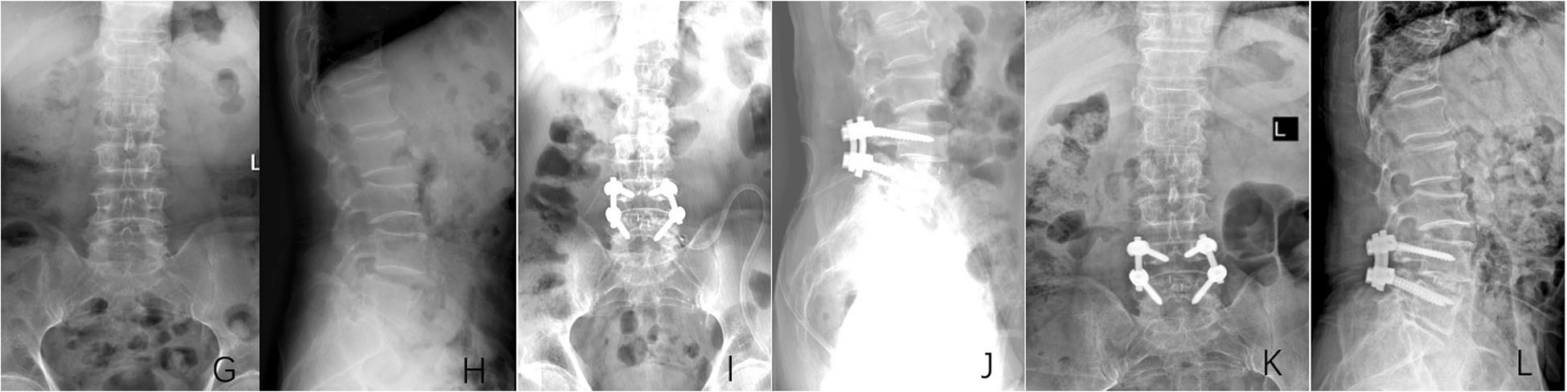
A 69-year-old male patient suffered from low back and left lower limb pain for 2 years, aggravating for 1 month. X-ray of the lumbar spine showed a typical case of single-level PLIF for L4 degenerative spondylolisthesis. g Preoperative anteroposterior radiograph. h Preoperative lateral radiograph. i Postoperative anteroposterior radiograph. j Postoperative lateral radiograph. k The last follow-up anteroposterior radiograph. l The last follow-up lateral radiograph
